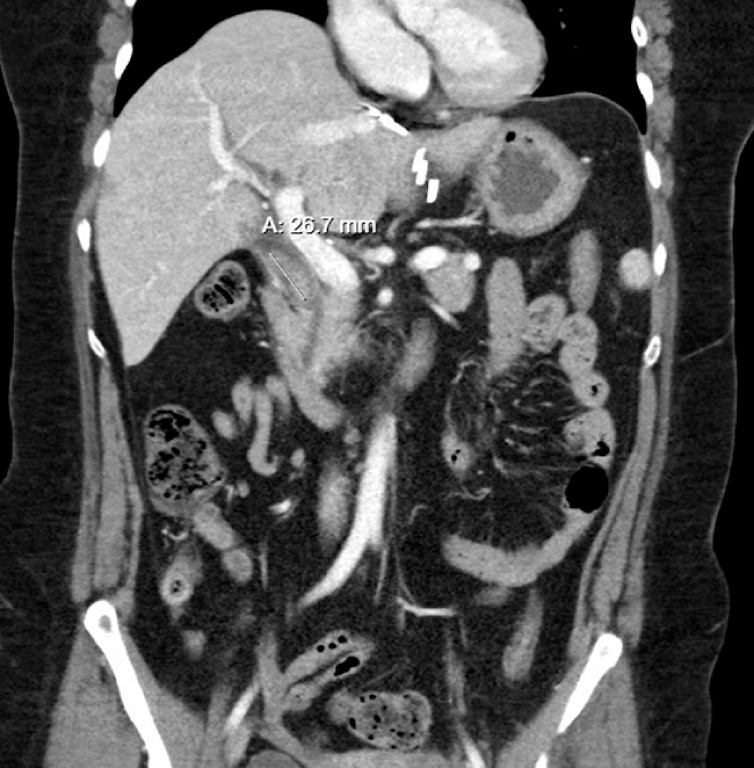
Initial computed tomography demonstrating 2.7-mm defect in the common bile duct.
